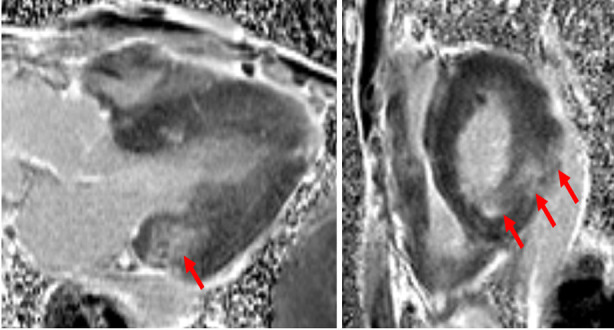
Cardiac magnetic resonance images of the same patient as in Fig.1. Left panel is vertical 3-chamber view and right panel parasternal short axis view demonstrating areas of LGE in the basal posterior and inferolateral walls (arrows). LGE, late gadolinium enhancement.
